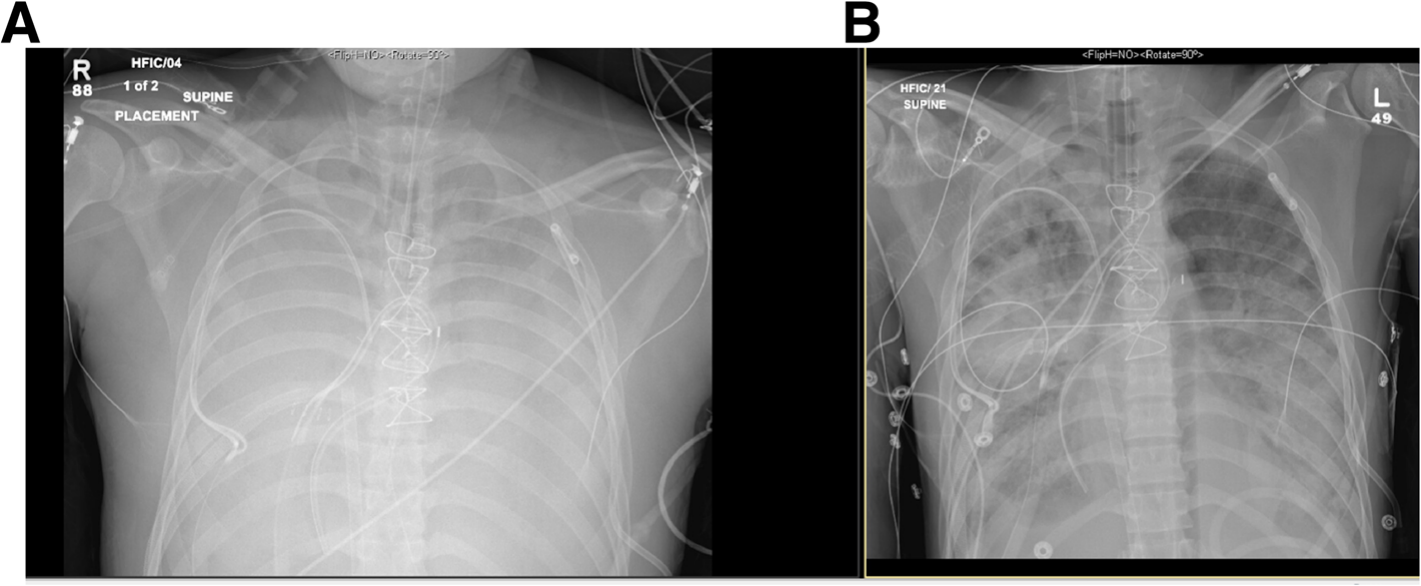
a Chest x-ray on admission to ICU one day after initial CXR at admission showing diffuse infiltrates with loss of lung volumes. b Improvement in aeration noted in four days after cannulation with ECMO
